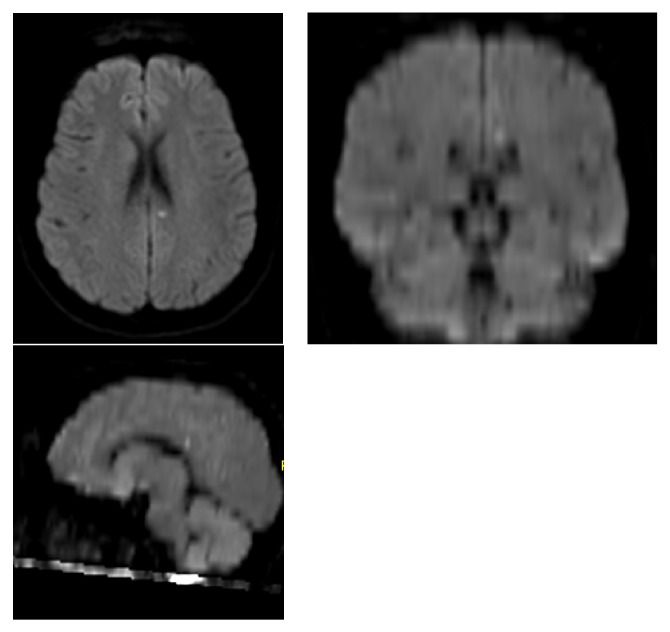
DWI in axial, coronal, and sagittal views (Siemens Aera 1.5T) (ST 5mm, TE 16ms, TR 5230ms) showing a single diffusion abnormality in left cingulate gyrus.
